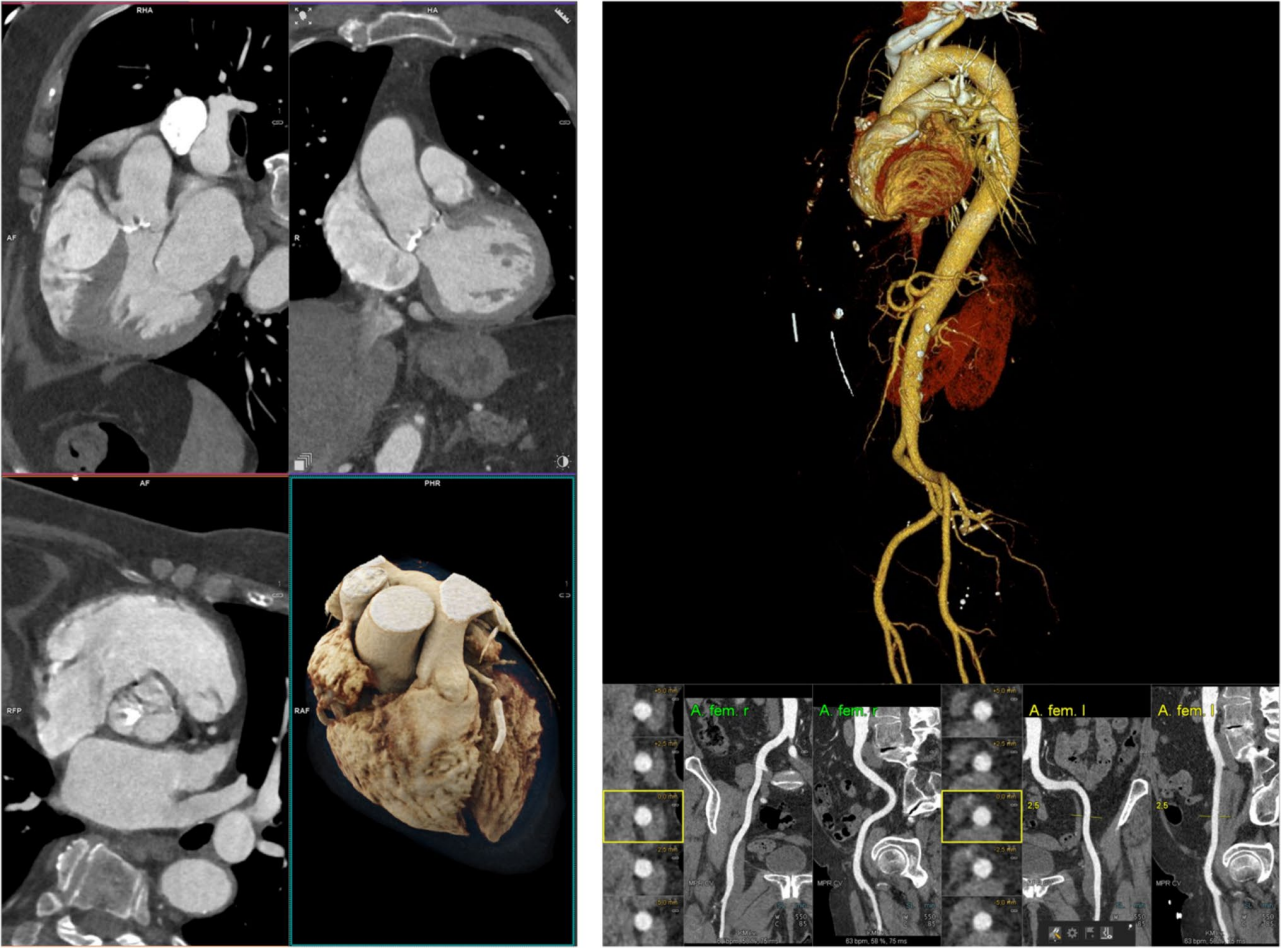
Transcatheter aortic valve replacement (TAVR) planning using CT. The images on the left delineate the measurement of the aortic annulus prior to TAVR in 3 different orientations of the aortic valve. The right image shows the access path via the right (A. fem. r) and left femoral artery (A. fem. l)
